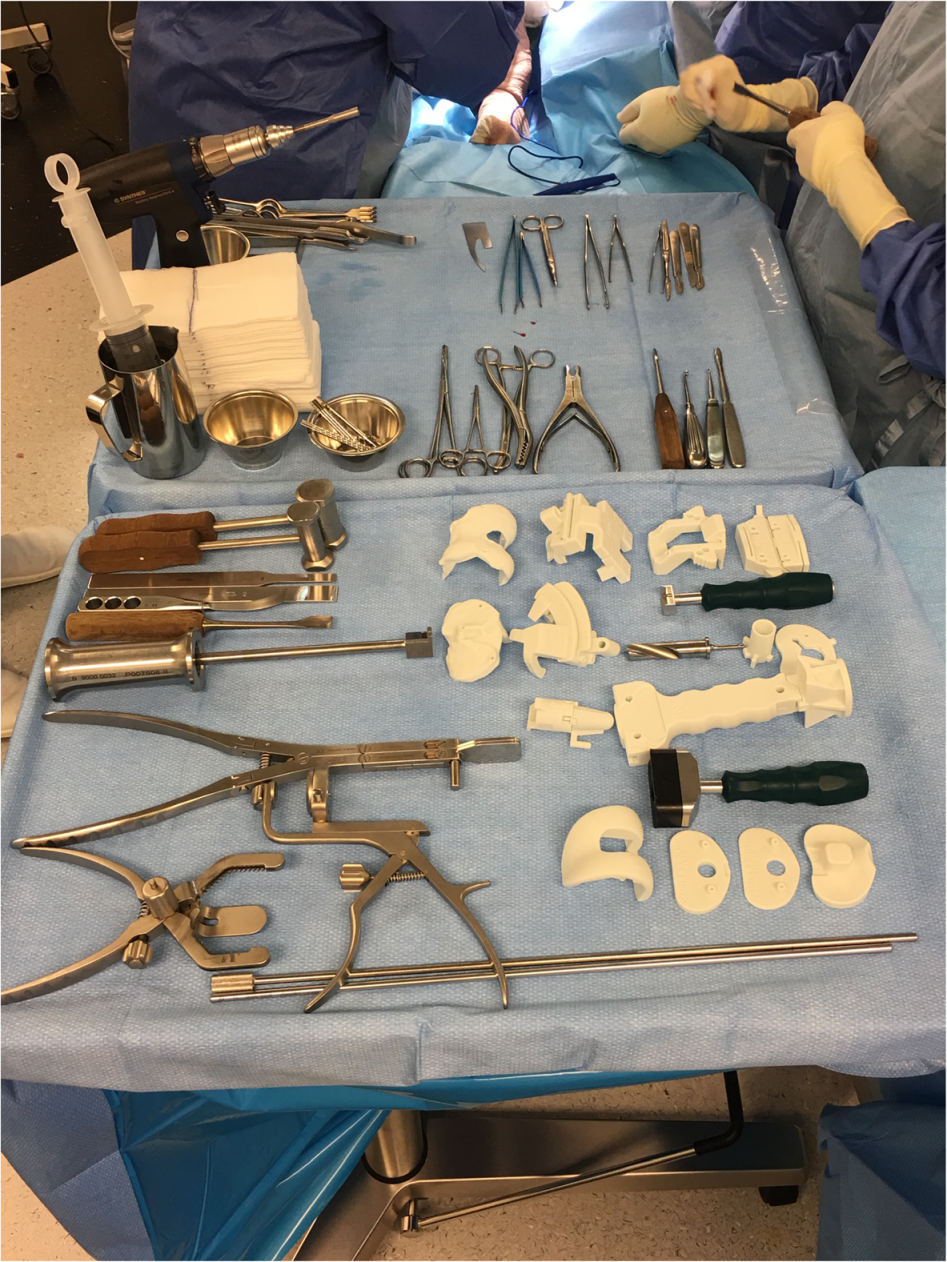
Two surgical instrument trays in the operating room. The upper tray contains standard metal instruments. The lower tray contains standard metal instruments and the different CT-based, patient-specific, single-use instruments, ancillary and cutting guides (Knee-Plan® Set), shown in white. Single-use material in first (top) row, from left to right: femoral model, distal femoral cutting guide, intercondylar cutting block, 4-in-1 cutting block. Second row: tibial model, tibial cutting guide, tibial drill guide, tibial trial. Third row: tibial stem trial, impactor with impaction pad. Fourth (bottom) row: femur trial, 2 trial insert augments (+2mm and +5mm), trial insert
